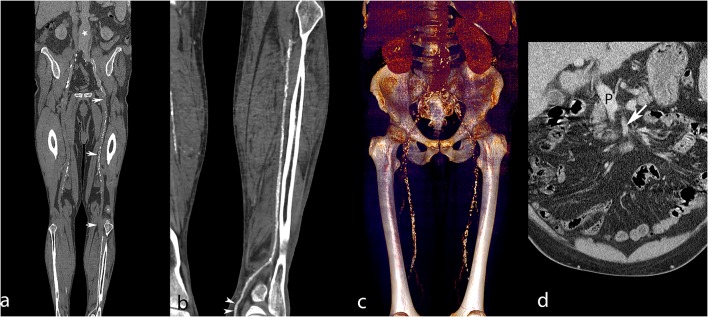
Lower extremity CT angiography obtained with the RA technique in a 65-year-old man with a history of ischemic and hypertensive heart disease, atrial fibrillation, chronic obstructive pulmonary obstruction, past stroke, and an aneurysm of the abdominal aorta. The patient was admitted for critical ischemia of the lower limbs, characterized by Fontaine stage IV disease of the left lower limb with superficial ulceration. Curvilinear reconstruction of the left aortopedal arterial system (a) shows inadequate enhancement of the abdominal aorta (white star) and absent enhancement of the superficial femoral artery due to an extended occlusion (white arrows). Curvilinear reconstruction of the left posterior tibial artery (b) shows optimal enhancement till the pedal level (white arrowheads). Volume rendering reconstruction of the abdominal aorta and femoral arteries (c) provides limited visualization of the arterial system and shows enhancement of the liver and kidneys related to venous contamination. Coronally reformatted slice through the abdomen (d) shows enhanced portal vein (P) and insufficiently enhanced superior mesenteric artery (white arrow), meaning that the sampling instant was too late for arterial phase imaging. Attenuation measurements were as follows: aortic level, 99 HU; iliac level, 85 HU (right) and 94 HU (left); femoral level, 123 HU (right), and 168 HU (left); tibial level 270 HU (right), and 305 HU (left); pedal level, 235 HU (right), and 273 (left). HU, Hounsfield units.
